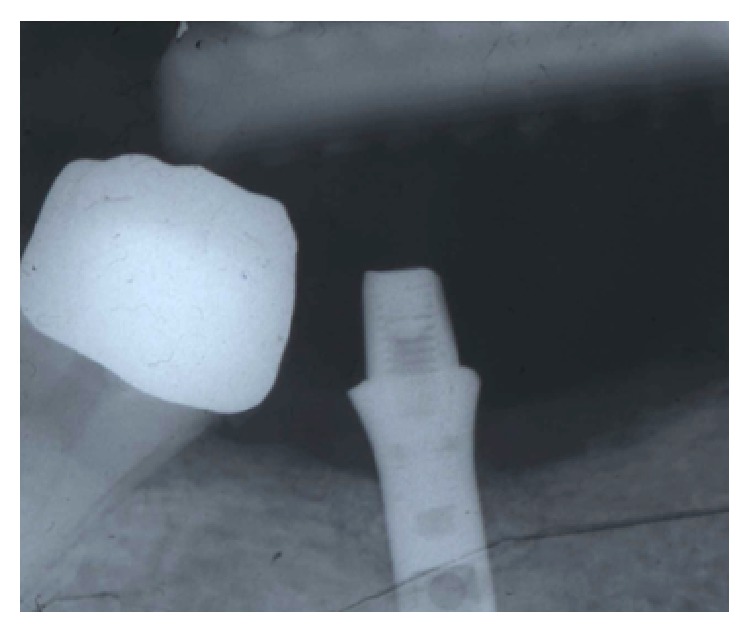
Postoperative intraoral radiography. The graft filled into the bone defect was observable in close contact with the implant surfaces. During the healing period the implant was left unloaded.
